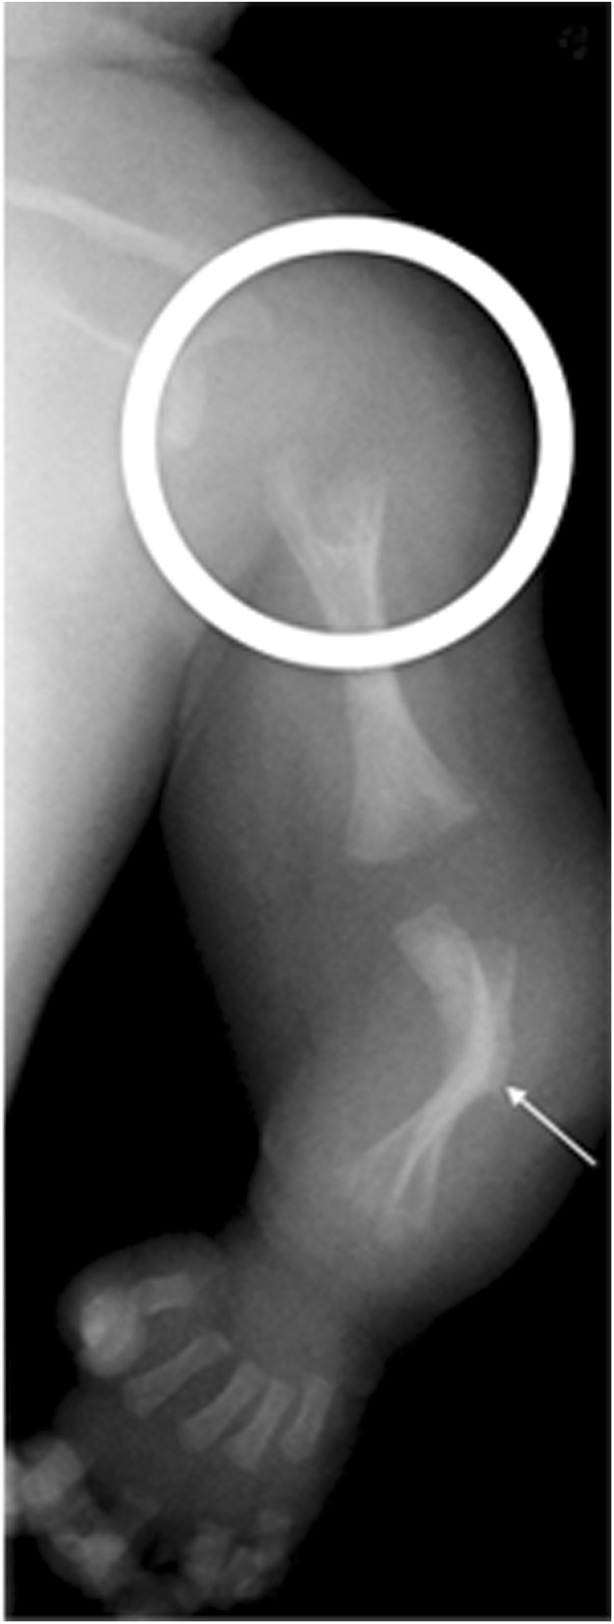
Imaging features of hypophosphatasia of an infant of unknown gender at birth. Anteroposterior radiograph of the left upper limb shows metaphyseal “tongues” of radiolucency of the left proximal humerus (*circled*), with bowing and spurring (*arrow*) of the radius and ulna. [Image reproduced with permission from Radcliffe Publishing [38], page 374, case 11, image 11c]
